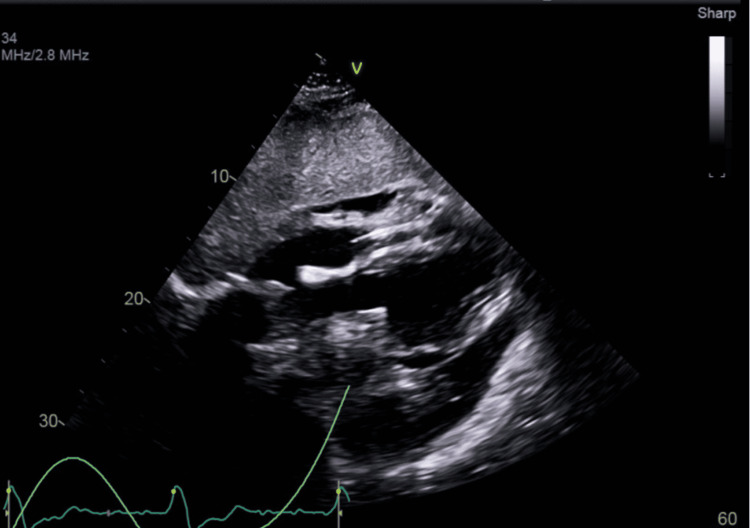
A small pericardial effusion and apparent diastolic collapse of the right ventricle seen on echocardiogram
